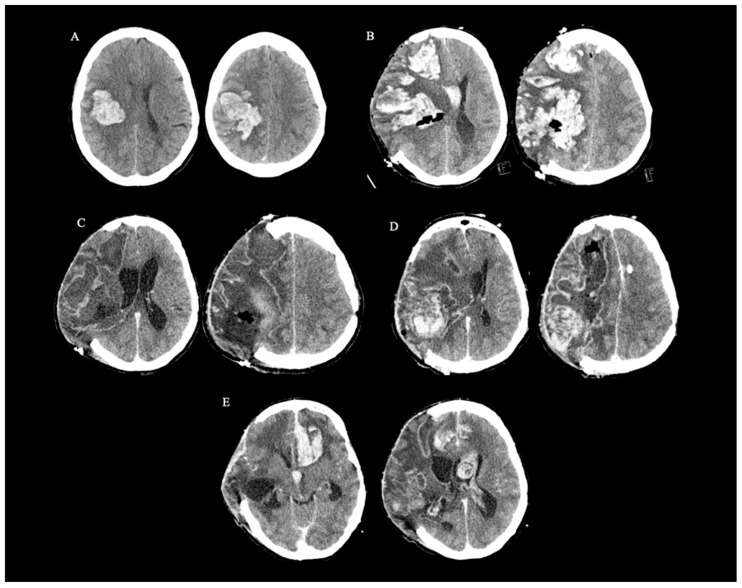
Head computed tomography (CT) scans of the patient at different time intervals. (A) at admission showing a 6 × 3 cm cortical—subcortical intracerebral haematoma (ICH) with surrounding cerebral oedema in the right temporoparietal lobe with subfalcine herniation. (B) Postoperatively showing a massive ICH in the right frontotemporoparietal region with haematocephalus, cerebral oedema and subfalcine herniation. (C) A month after admission (head CT scan with contrast) showing a 15 × 8 × 9 cm intracranial abscess in the right hemisphere. (D) Postoperatively (head CT with contrast) showing residual abscess with a recurrent ICH in the right parietal region with subfalcine, uncal and descendent transtentorial herniation. (E) Two months after admission showing a new ICH in the left frontal region and blood in the left lateral ventricle.
